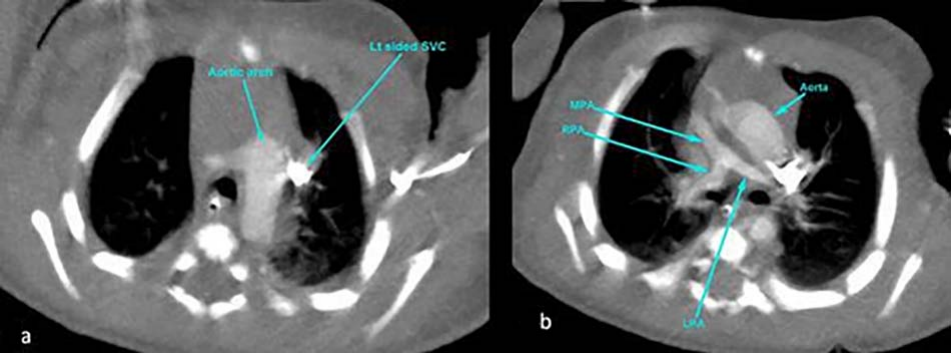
CT angiography thorax showing the left-sided aortic arch (a) and inverse relationship between ascending aorta and pulmonary artery. The MPA origin is atretic with hypoplastic RPA and LPA compared with the aorta (b). SVC, superior vena cava; RPA, right pulmonary artery; LPA, left pulmonary artery.
